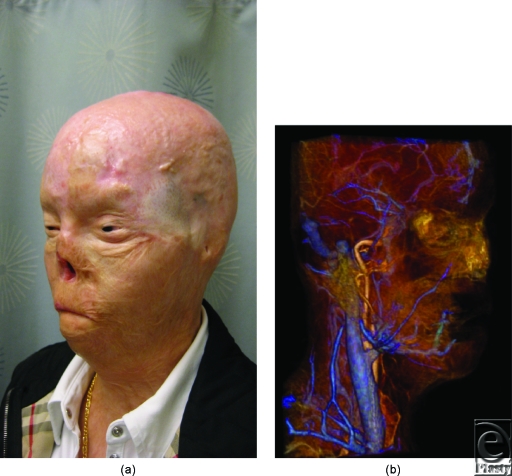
(*a*) Candidate 3 during initial evaluation for face transplantation. (*b*) Venous phase conventional magnetic resonance (MR) venography. In patients without artifact in close proximity to the major vessels considered for anastomoses, both computed tomography and MR imaging demonstrate excellent venous detail.
